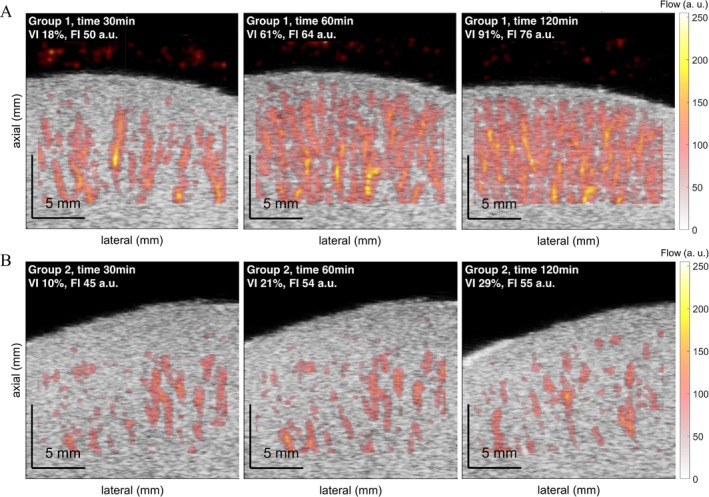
Representative Power Doppler (PD) imaging frames of renal cortex in (A) Group 1 and (B) Group 2 at 30, 60, and 120 min of NMP. FI, flow index; VI, vascularization index. [Color figure can be viewed at wileyonlinelibrary.com]
